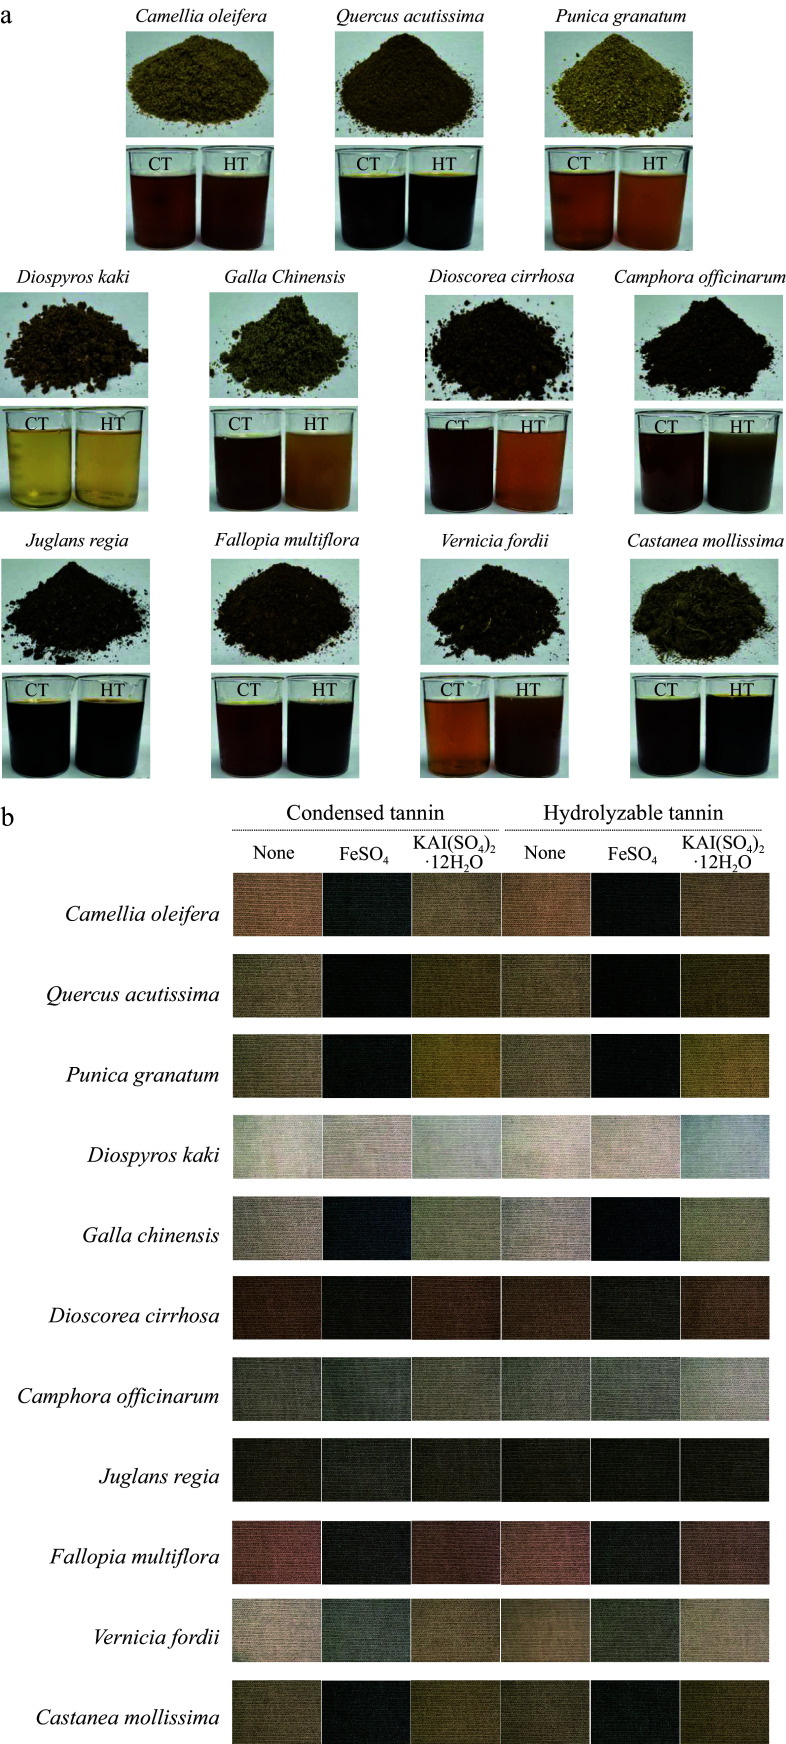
Plant dyes and dyeing results. (a) Photos of the powder and extracted dye solutions from the 11 plant dyes. CT refers to the dye solution extracted using 60% ethanol as the solvent, with condensed tannins as the main component; while HT refers to the dye solution extracted using water as the solvent, with hydrolysable tannins as the main component. (b) Dyeing results of cotton fabrics with different plant dyes and mordants.
